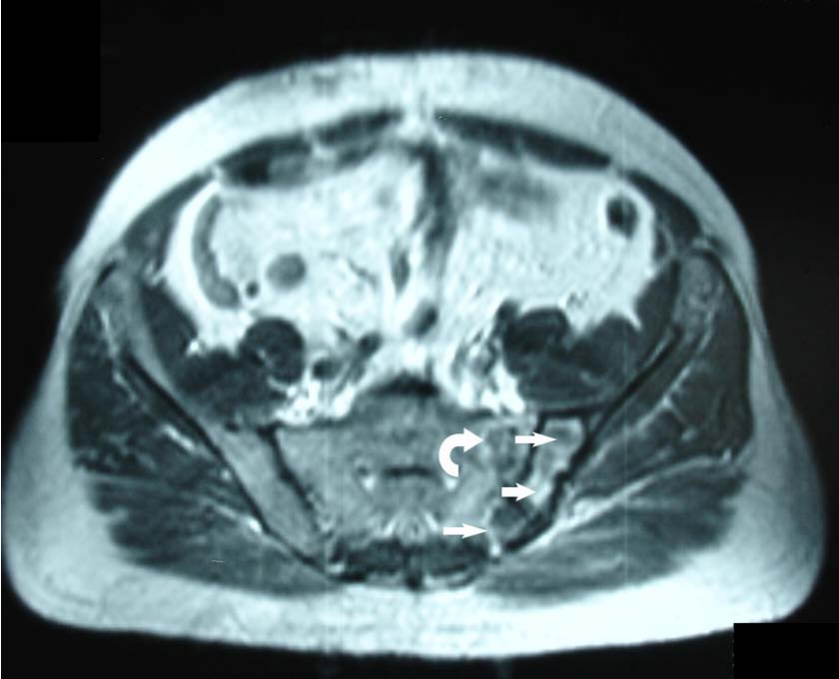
Post-contrast axial T1-weighted MR image shows the postoperative changes with diminution of the extension and the enhancement of the tumor at the left iliac bone (arrows) and at the left sacral wing (curved arrow).
